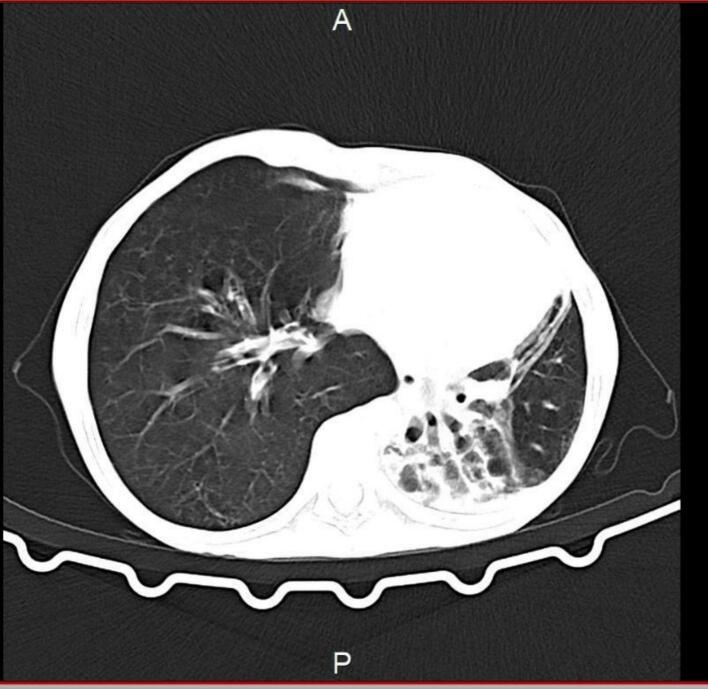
Axial chest CT shows severe volume loss and bronchiectasis of the left lung, with compensatory hyperinflation of the right lung—findings consistent with Swyer-James-MacLeod syndrome.
